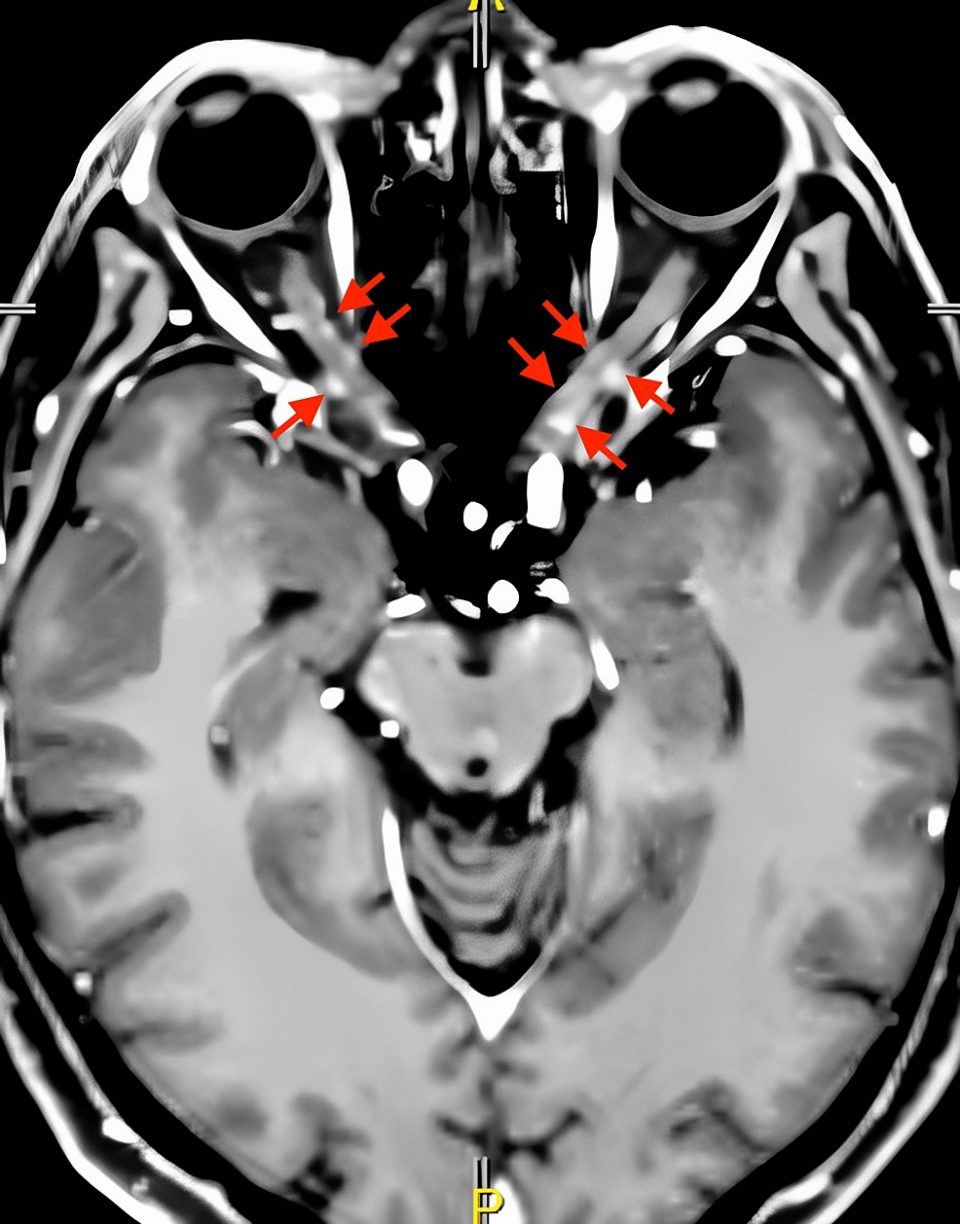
Post-contrast T1-weighted MP-RAGE study showing abnormal enhancement (red arrows) surrounding the bilateral optic nerves MP-RAGE: Magnetization Prepared–RApid Gradient Echo.
